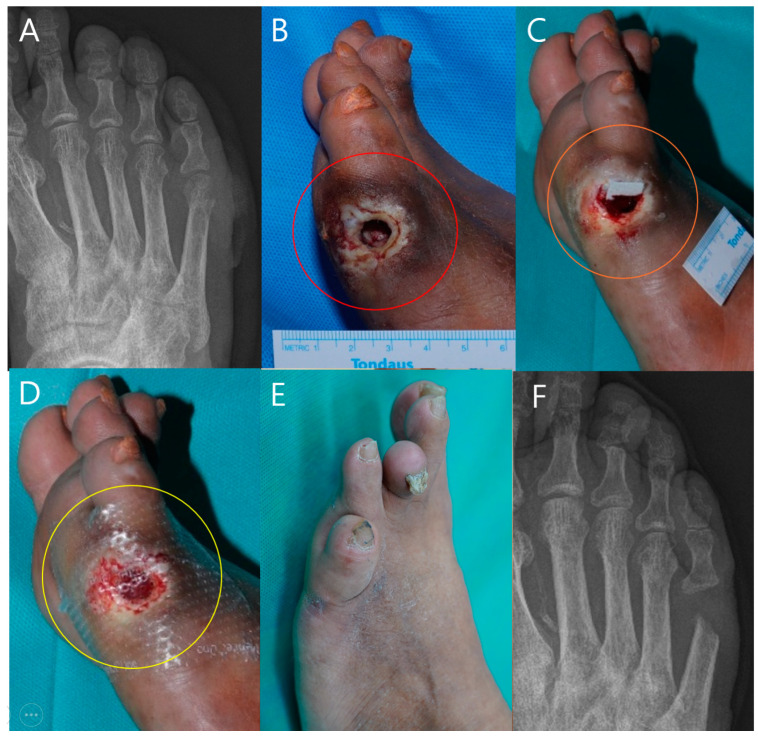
A 65-year-old male patient with a history of diabetes and peripheral arterial disease. (A) Initial X-ray showing the extent of bone involvement. (B) Clinical photograph prior to debridement, highlighting the necrotic tissue and bone exposure (red circle). (C) Post-debridement image showing the wound bed before micronized acellular dermal matrices (mADMs) application (orange circle). (D) Wound after 3 weeks following a total of five mADM applications, demonstrating significant granulation and healing progress (yellow circle). (E) Wound at the 8-week follow-up, showing re-epithelialization. (F) Final X-ray showing successful healing despite the presence of a bone defect, indicating effective re-epithelialization and wound closure.
